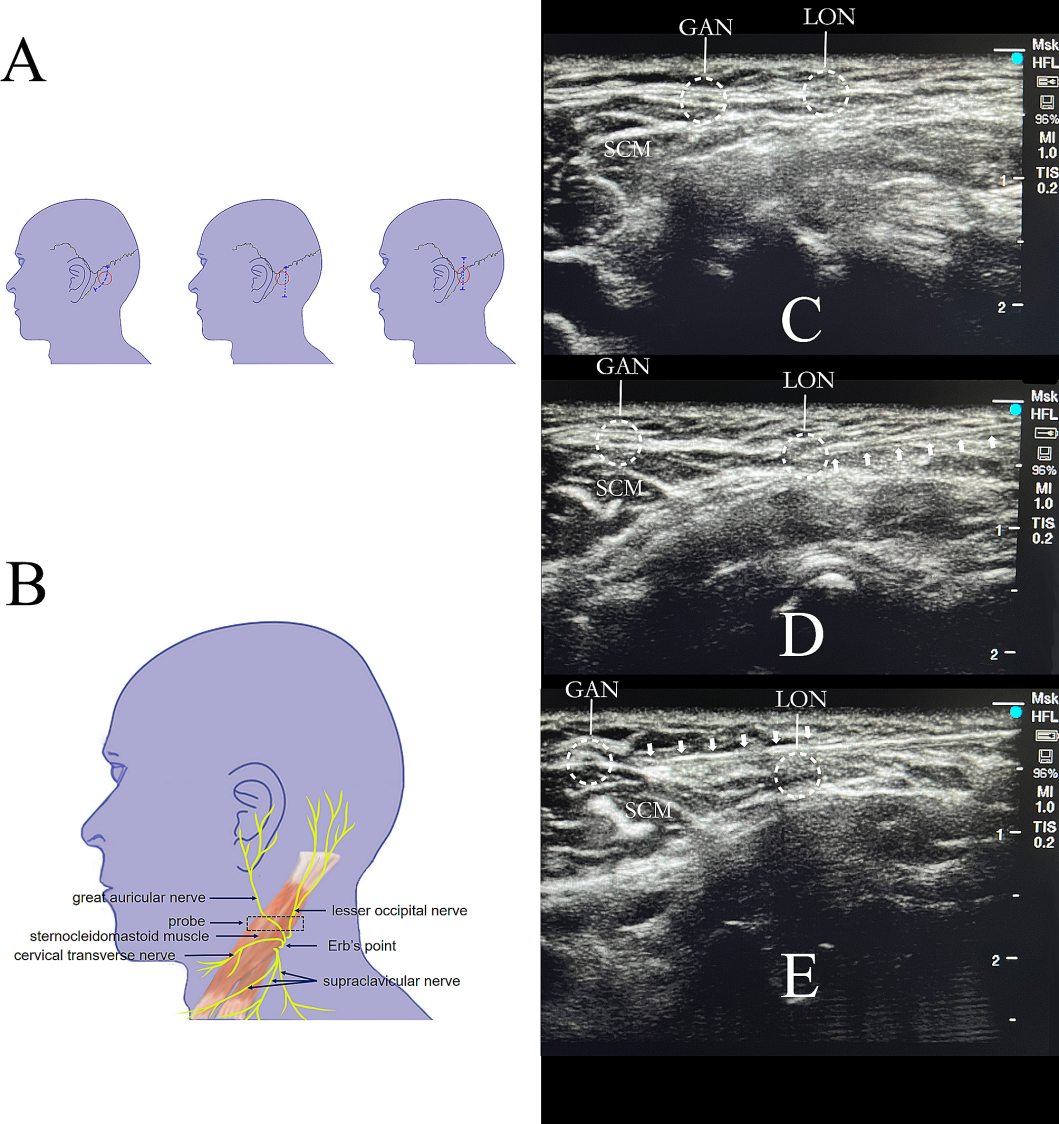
Surgical incisions, anatomy of cervical plexus and ultrasound-guided lesser occipital nerve combined with great auricular nerve block. (A) Three types of posterior suboccipital retrosigmoid incisions for vestibular schwannoma craniotomy. The red circle indicates the tumor site, and the blue dashed line indicates the commonly used incisions. (B) Branches of the cervical plexus and placement of the ultrasound probe for the lesser occipital nerve combined with great auricular nerve block. (C) Ultrasound images of lesser occipital nerve and greater auricular nerve. (D) Ultrasound-guided lesser occipital nerve block. (E) Ultrasound-guided greater auricular nerve block. GAN: greater auricular nerve; LON: lesser occipital nerve; SCM: Sternocleidomastoid muscle. The white arrows indicate the shaft of block needle
